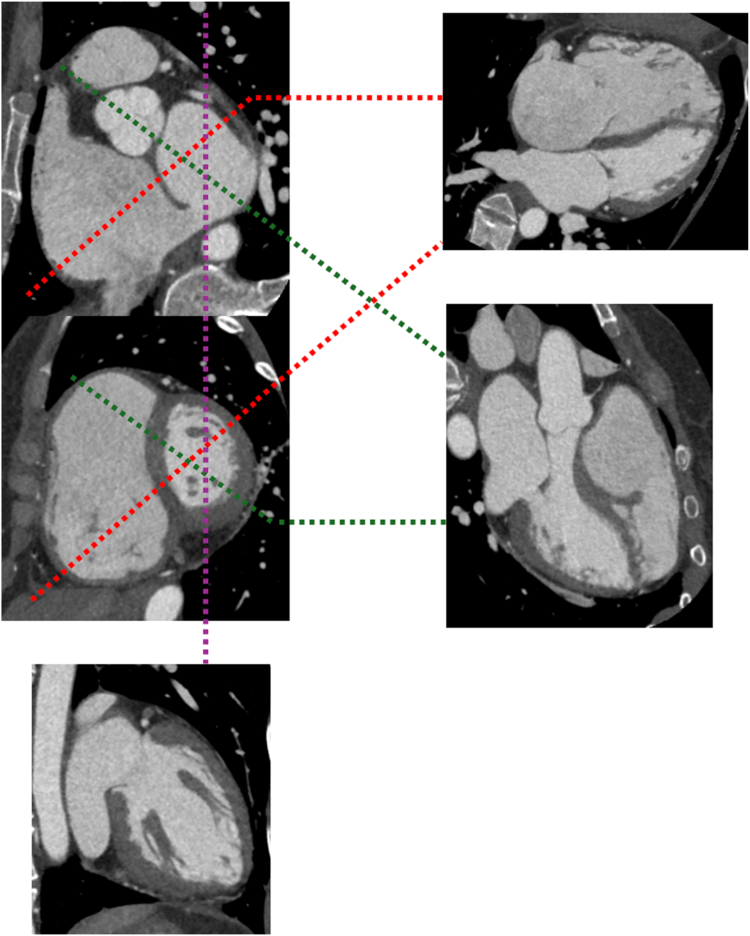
Cardiac computed tomography in multiple different planes (*red line*: apical 4-chamber; *green line*: apical 3-chamber; *purple line*: apical 2-chamber) demonstrates that the 2-chamber view best demonstrates the unroofed CS.
